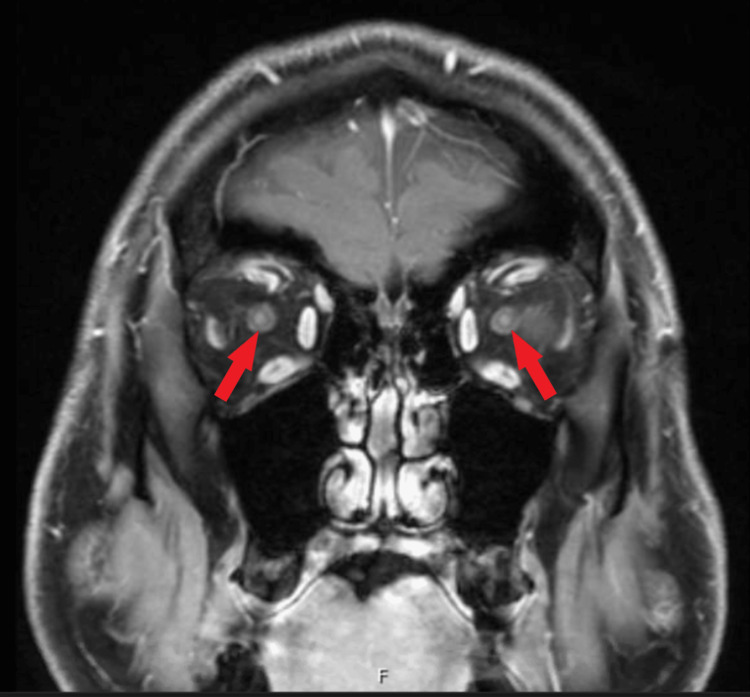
MRI of the orbits with contrast showing subtle uniform enhancement of the left and right optic nerves immediately behind the globes (arrows)
